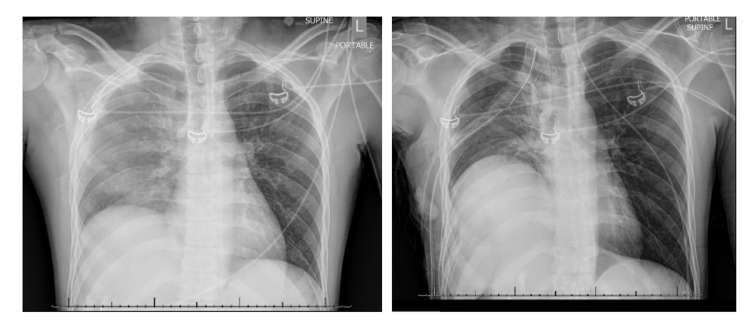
Chest X-ray before (left) and after (right) tube thoracostomy placement with right-sided diaphragm elevation.
